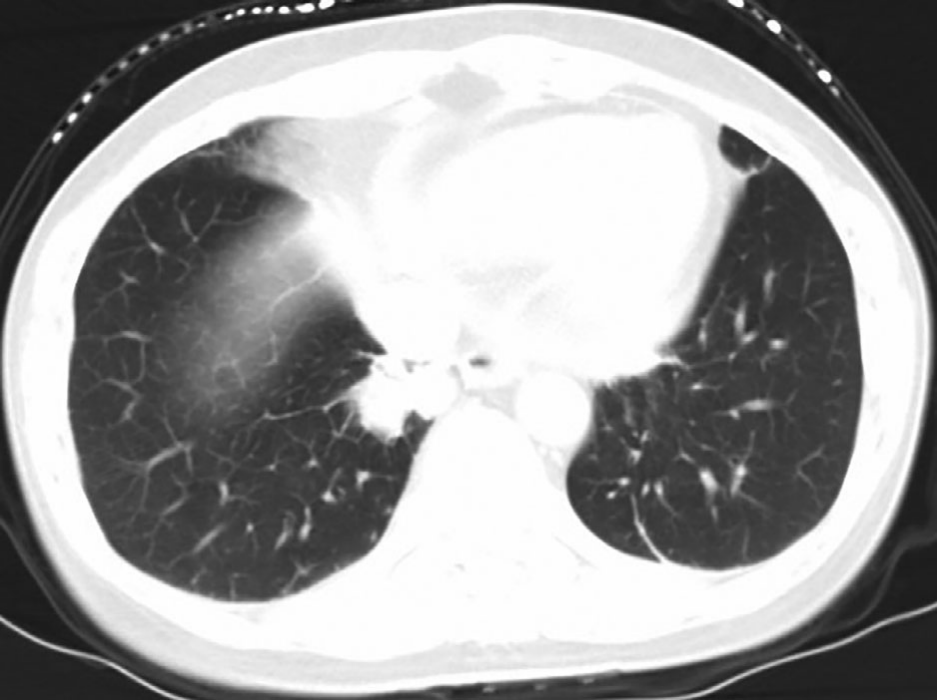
Chest computed tomography (CT) scan revealed a 2.6 × 2.3 cm nodule in the right inferior lobe of patient No. 5.
